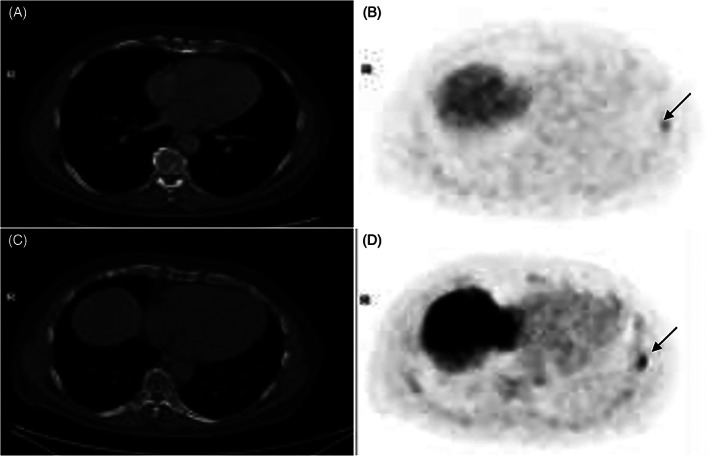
Two PET/CT scans of 72‐year‐old patient with known prostate cancer (Gleason 4 + 3, PSA 5.5) performed with F^18^PSMA (lower row) and 2 month later with Ga^68^PSMA (upper row). Focus (arrow) of increased uptake (b, d) visualized in both scans in the seventh left rib without significant interval changes (slightly less prominent intensity of uptake on the Ga^68^PSMA scan in comparison with the F^18^PSMA). Negative for prostate cancer results obtained from biopsy of this rib
